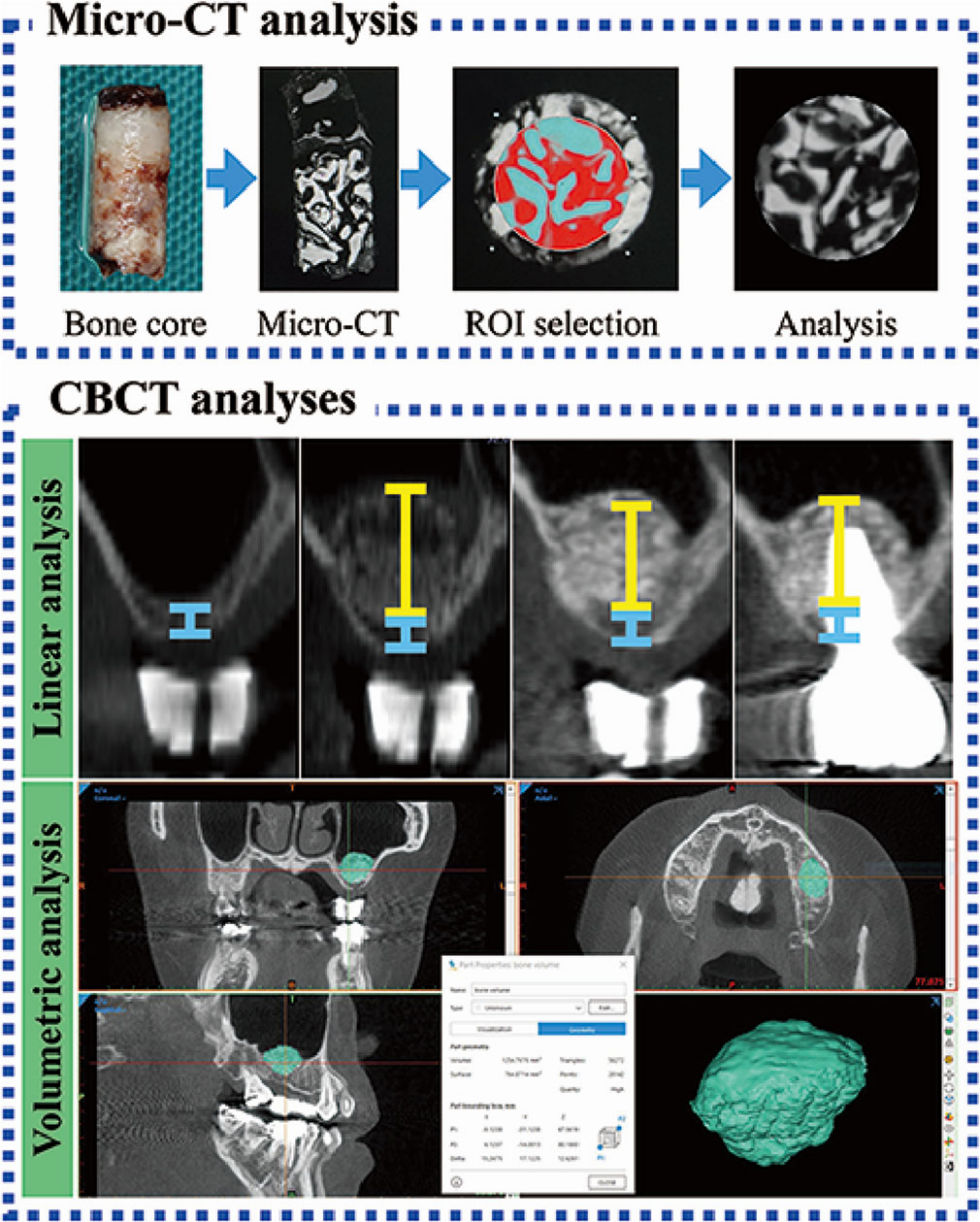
Microcomputed tomographic and conebeam computed tomographic analyses. Blue and yellow lines indicate residual native bone and augmented bone heights, respectively.
